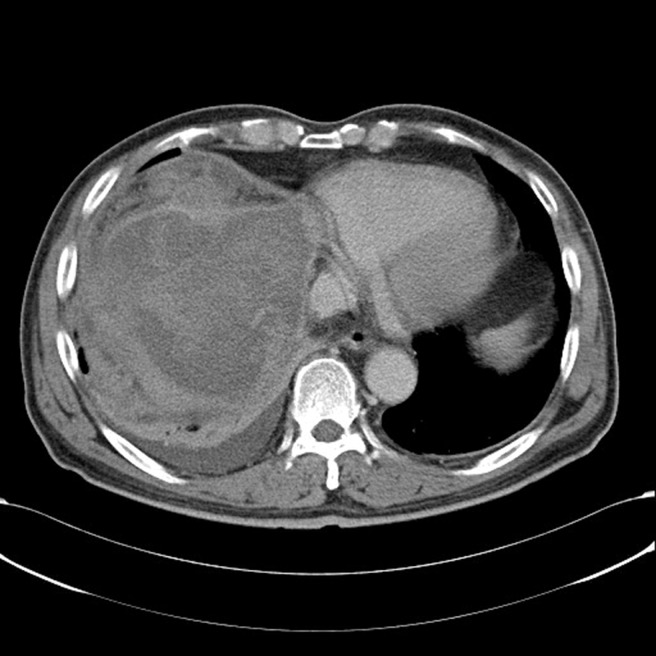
Abdominal computed tomography showed a huge, ruptured hepatocellular carcinoma with haemoperitoneum.
